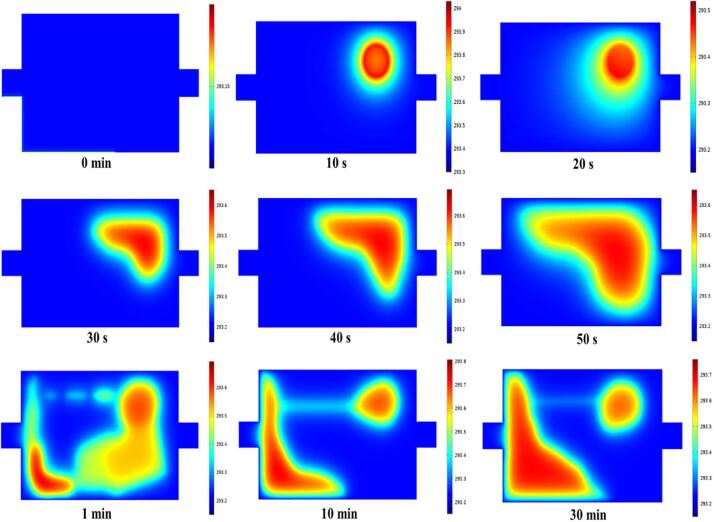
Temperature (K) field distribution under ultrasonic excitation for 0–30 min.
